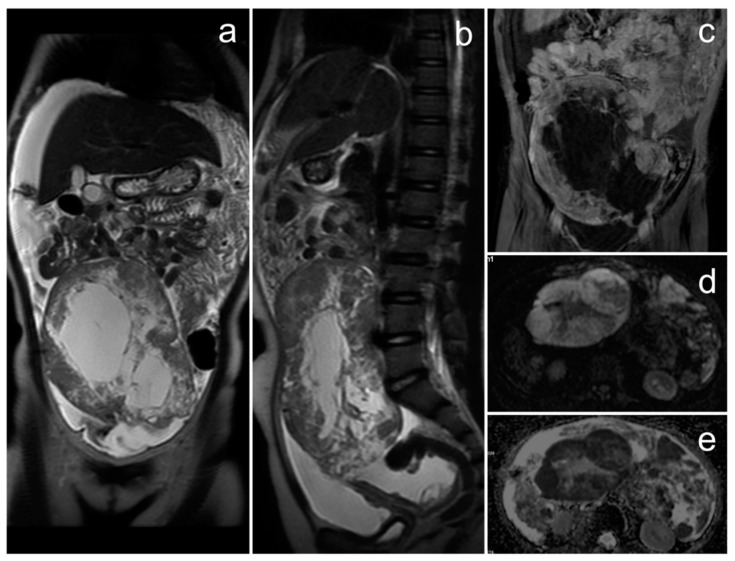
The MRI of a patient with small cell carcinoma of the ovary, hypercalcemic type (a–e). (a,b)—FSE/T2; (c)—post-contrast LAVA/T1 WATER; (d)—DWI; (e)—ADC map.
